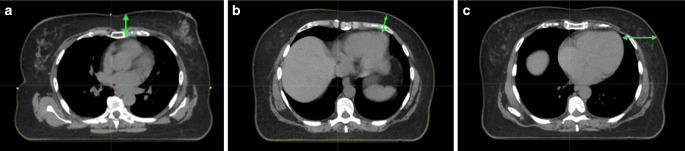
Axial CT slices with measurements (green arrows) of the a medial distance between the heart and the medial chest wall, b lateral distance between the heart and the lateral chest wall, and c mediolateral distance between the outer heart contour and the chest wall between the medial and lateral distance
